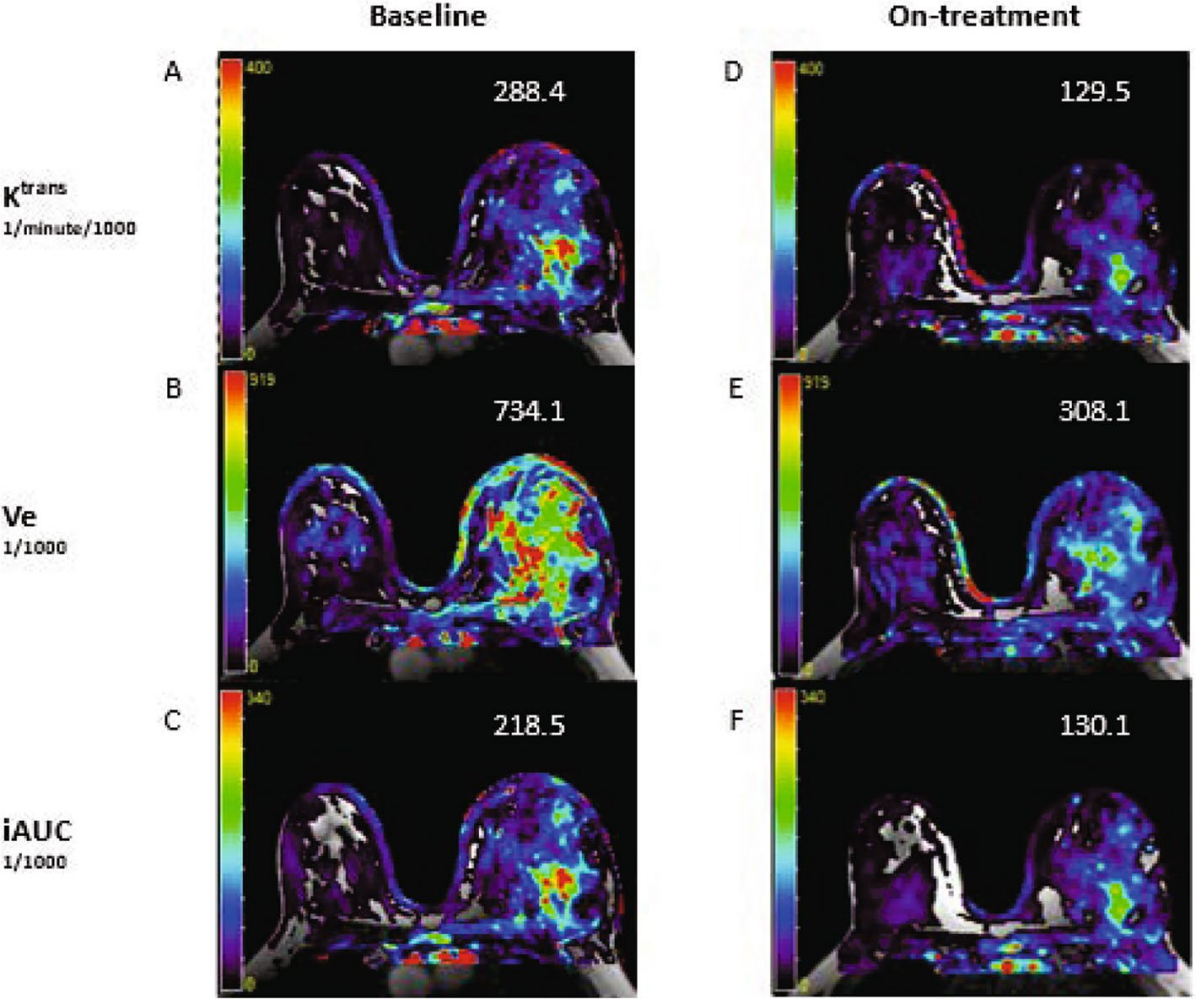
Example of DCE-MRI with decrease in K^trans^ (volume transfer constant from blood plasma to extravascular extracellular space), V_e_ (fractional volume of extravascular extracellular space), and iAUC (incremental area under the signal time intensity curve) on Day 8 on treatment compared to baseline. A 54-year-old woman with left inflammatory breast cancer (IBC) showed decrease in vascularity on treatment compared to baseline. A) Baseline K^trans^ B) Baseline V_e_ C) Baseline iAUC D) On-treatment K^trans^ E) On-treatment V_e_ F) On-treatment iAUC. DCE-MRI, dynamic contrast-enhanced magnetic resonance imaging
